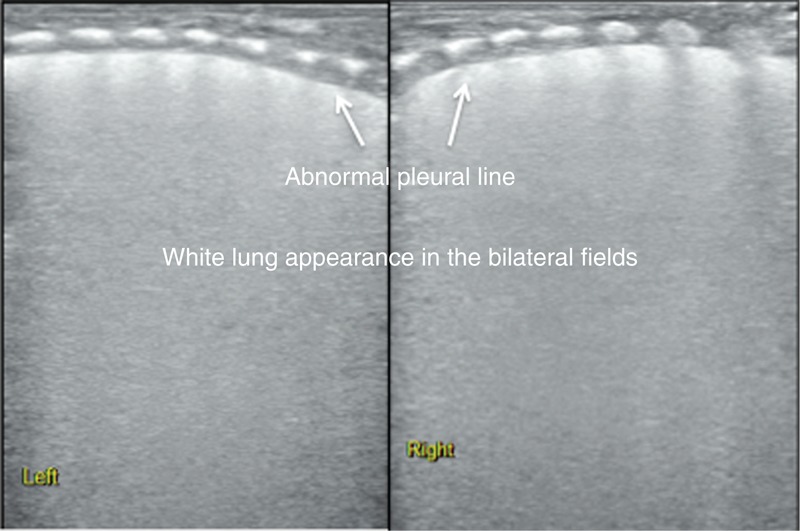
Ultrasonic manifestation of TTN: interstitial lung syndrome and white lung. The infant above had a GA of 27^+3^ wk and was delivered vaginally; the infant had a birth weight of 1020 g. The infant was admitted to the hospital after suffering from dyspnea for 6 h and was diagnosed with TTN based on an arterial blood gas analysis and CXR manifestations. Lung ultrasonography showed thickening and fuzziness of the pleural lines in both the lungs as well as A-line disappearance and white lung appearance. CXR = chest radiography, GA = gestational age, TTN = transient tachypnea of the newborn.
